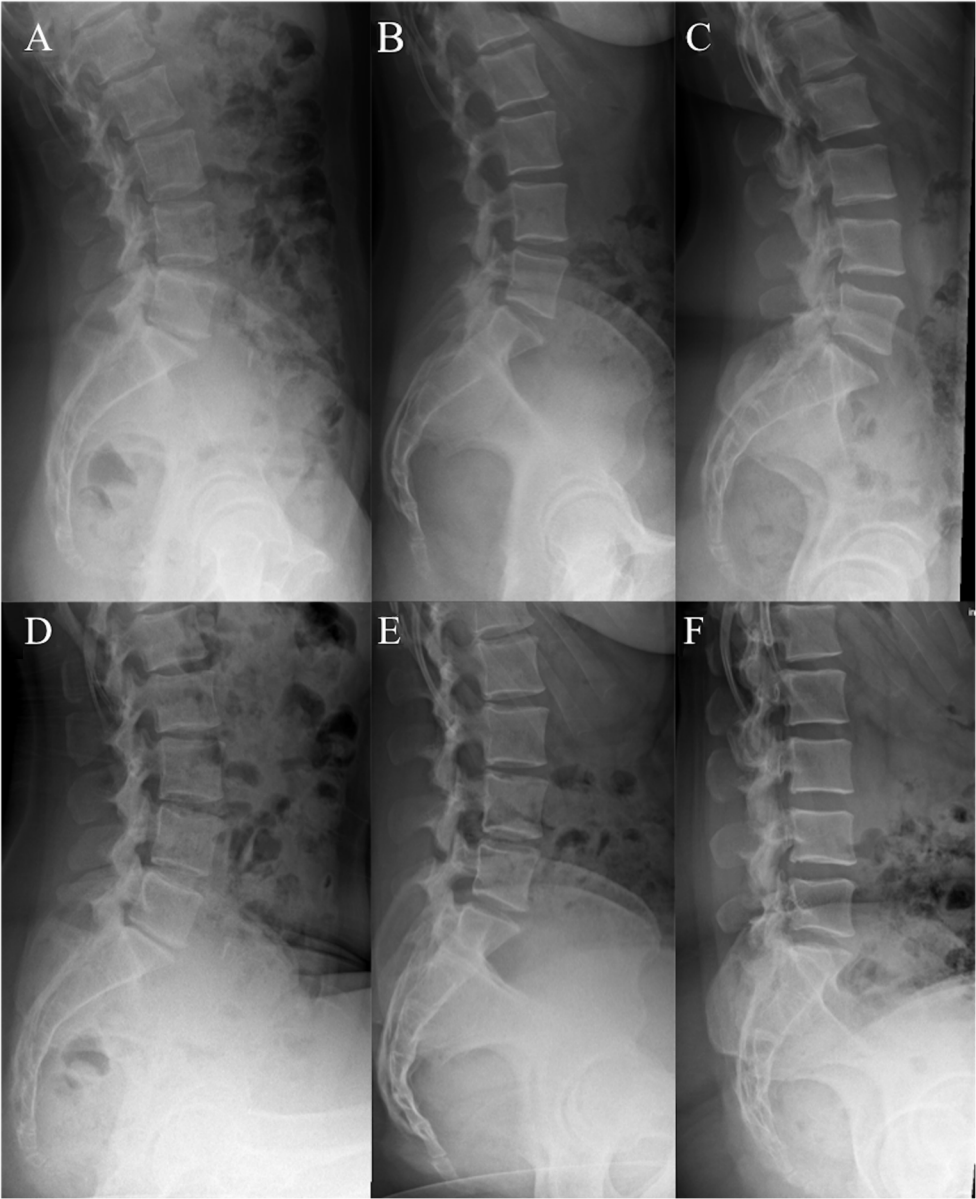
Representative radiographs with different types of functional lumbopelvic alignment in standing and relaxed-seated position (A/D Stiff - Δ PT < 10°, B/E Normal - Δ PT 10° − 30°, C/F Hypermobile - Δ PT > 30°). PT pelvic tilt
